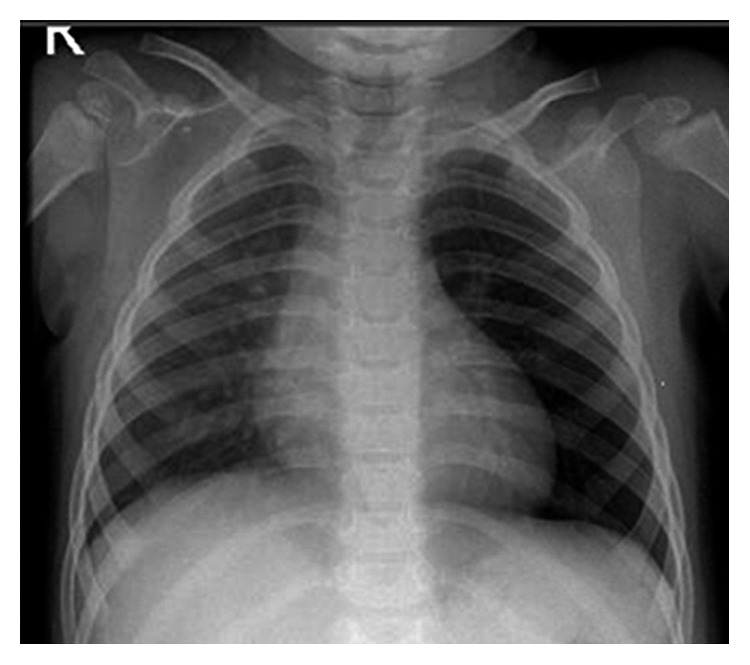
Chest X-ray showing hyperinflation on the left side.
